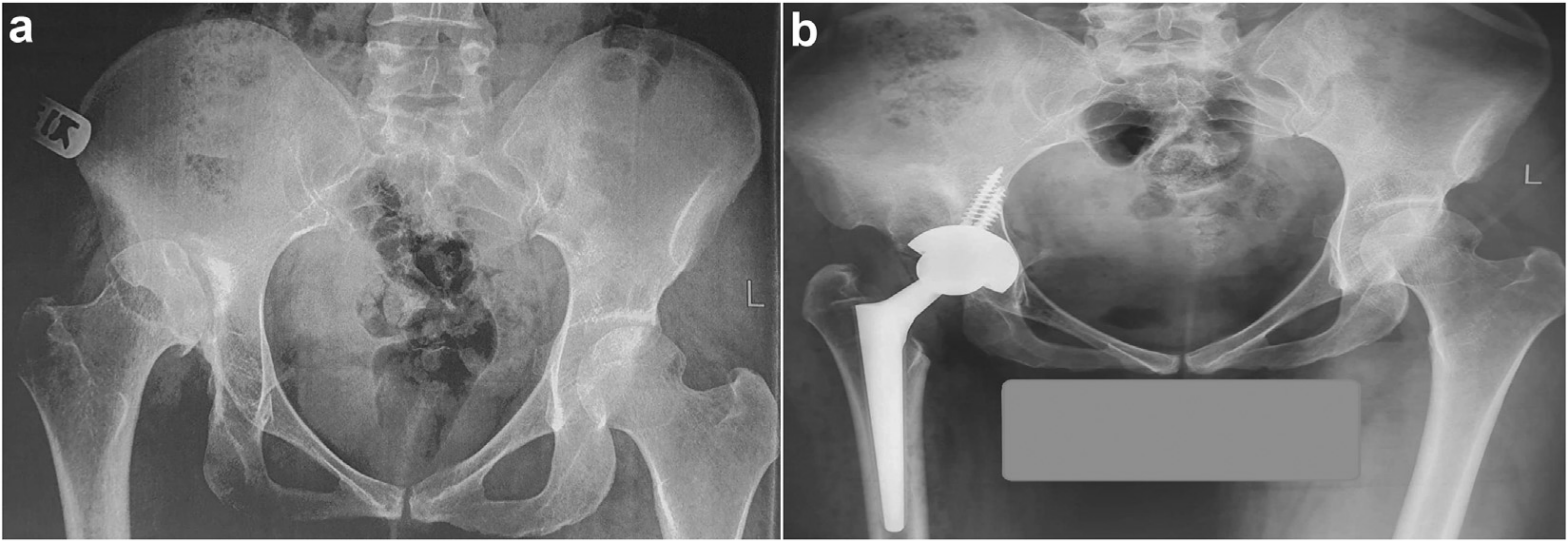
(a) Preoperative and (b) postoperative imaging of a patient with developmental dysplasia of the hip (DDH). In this case, trochanteric osteotomy was unnecessary due to the absence of neurological issues during intraoperative active neuromonitoring. Please note the equal length of both lower limbs in the postoperative X-ray.
